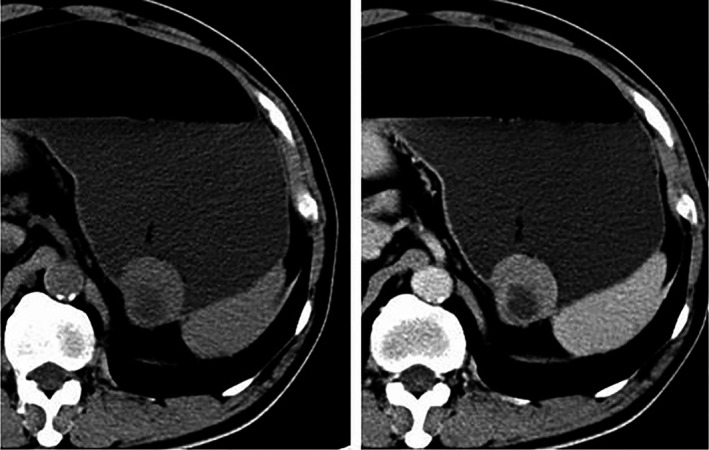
A 56‐y‐old man with a GIST in the stomach, low risk. Unenhanced and enhanced CT shows a 4‐cm mass with regular contour, well defined, necrosis, and moderate enhancement
